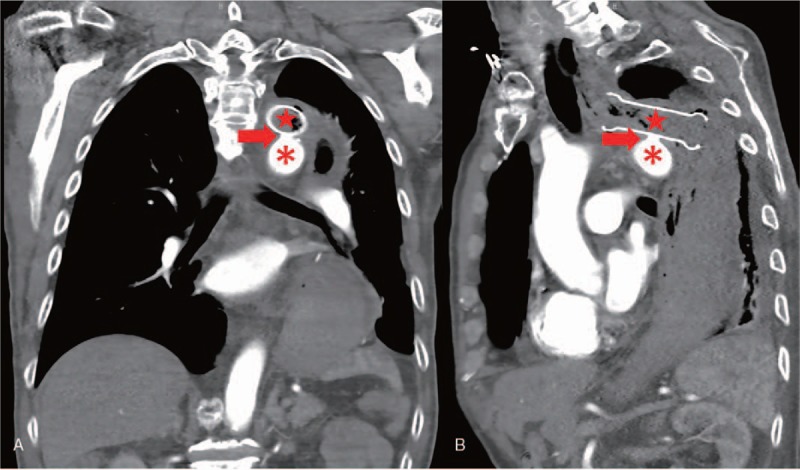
Computed tomographic angiography visualized an aortoesophageal fistula with an ulcer-like projection (red arrow head) on the aortic arch (red asterisk) where the esophageal stent (red star) touched. (A) Coronal position. (B) Sagittal position.
